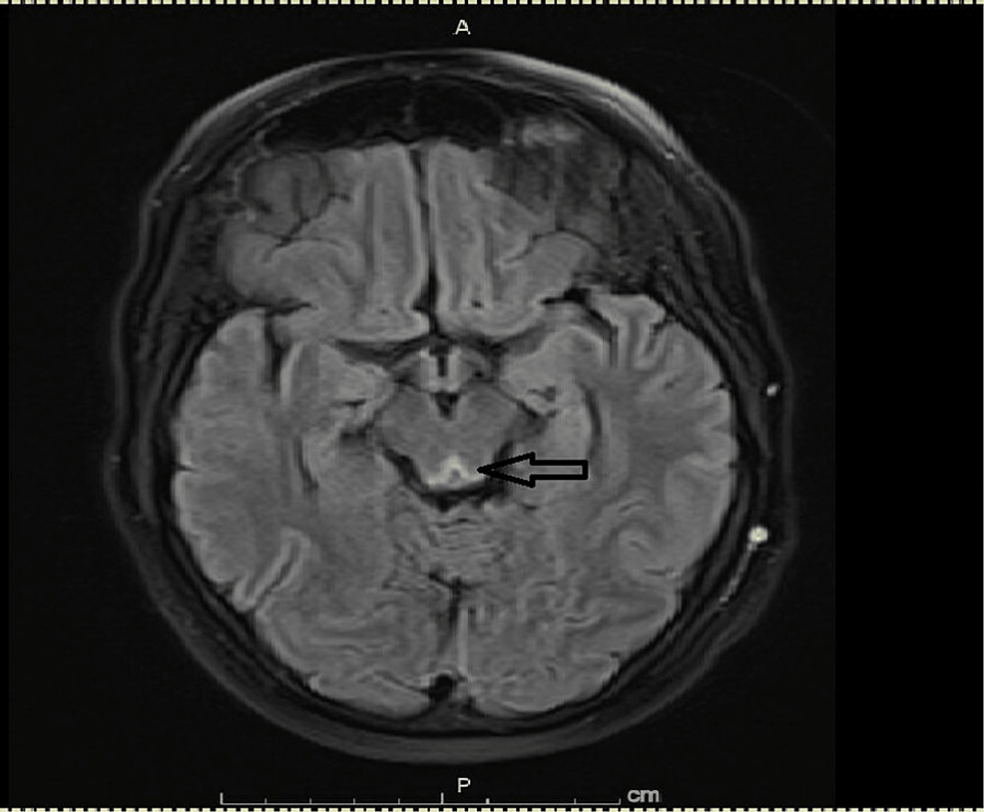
FLAIR MRI tectal hyperintensity FLAIR MRI of the brain shows hyperintensity over the tectum (arrow).
